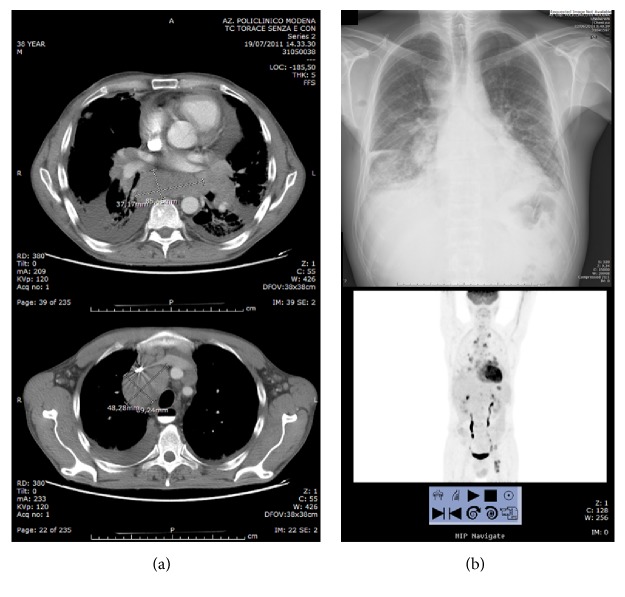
Radiological chest studies of our patient number 1. (a) Two scans of high-resolution CT, showing the mediastinal adenopathic masses. (b) Standard X-ray and total body PET scan, showing diffuse high-metabolism adenopathies.
